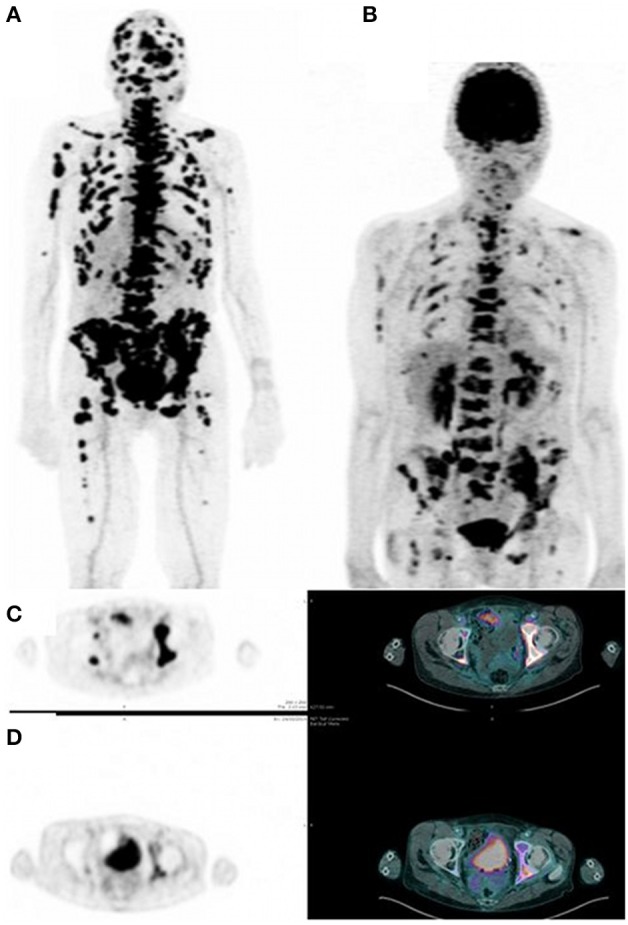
Pretargeted immuno-PET recorded after injection of 120 nmol of TF2 and 3 nmol of ^68^Ga-IMP-288 at 30 h in a patient with metastatic breast carcinoma. Immuno-PET (A,C) shows a high tumor uptake and a higher number of lesions as compared to FDG-PET (B,D).
